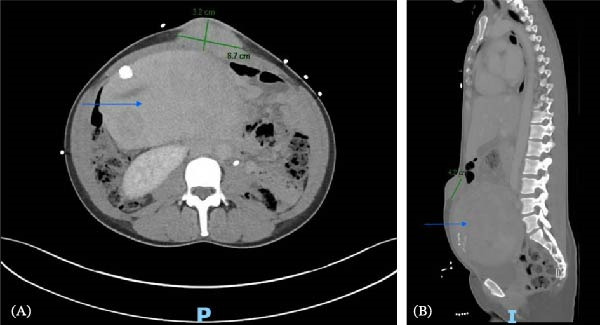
CT abdomen and pelvis depicting a ventral mass (marked in green) and a massively enlarged fibroid‐laden uterus (blue arrow) in (A) axial view and (B) sagittal view.
